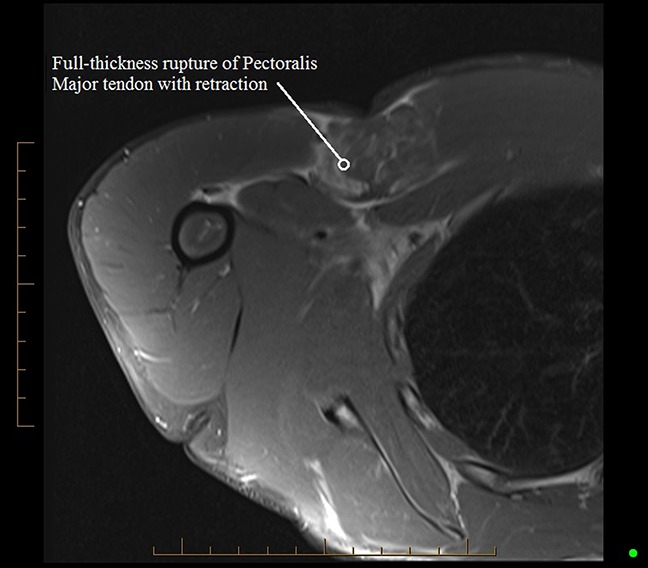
MRI proton density fat-suppressed axial scan right shoulder showing rupture of the pectoralis major tendon at the humeral insertion with 40-mm retraction.
